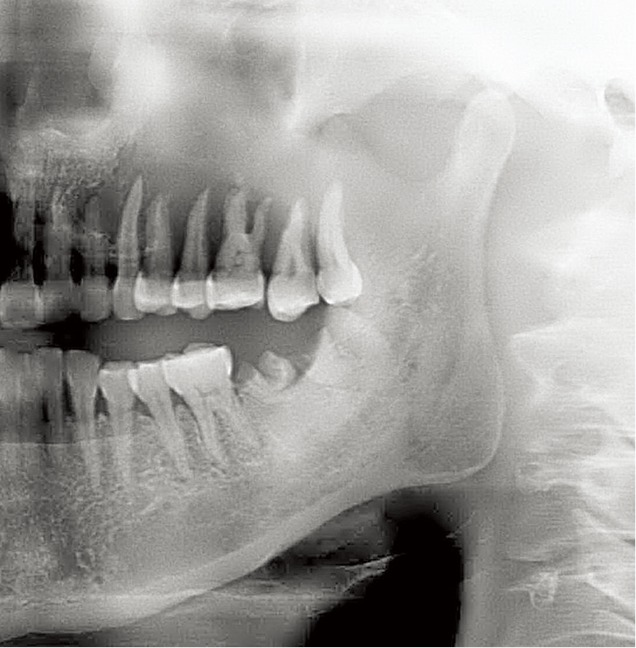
Panorex radiograph of a poorly defined, irregular osteolytic ACC lesion involving the left maxilla.
